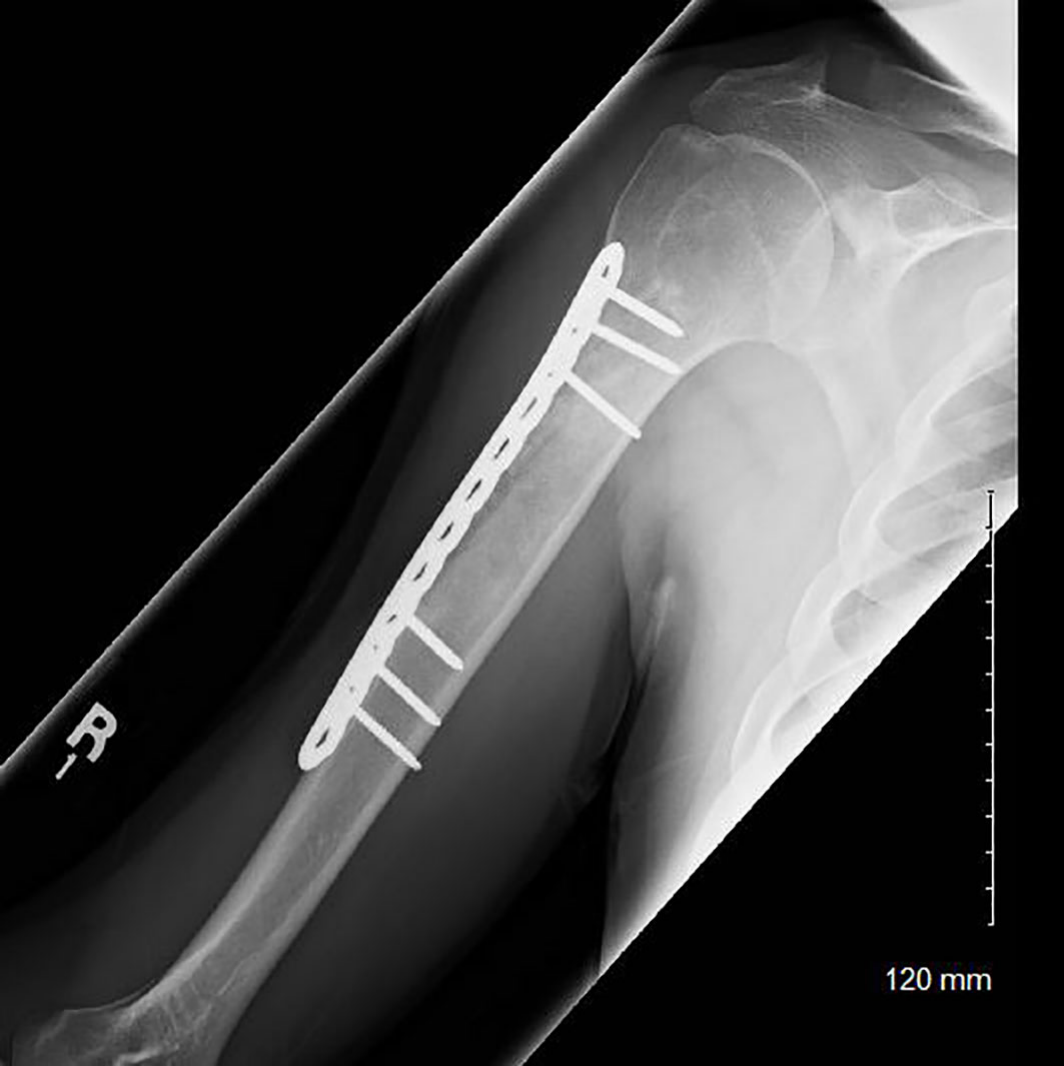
Post-operative radiograph of a patient who underwent curettage, grafting, and plating of a low-grade chondroid lesion of his proximal humerus.
